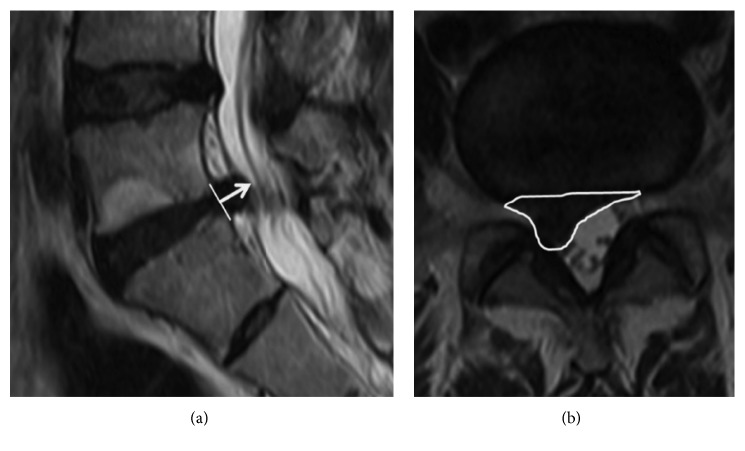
Protruded disc volume. The disc volume was calculated by multiplication of the height and the area. (a) The height (white arrow) in T2 sagittal MRI; (b) the area (white ellipse).
